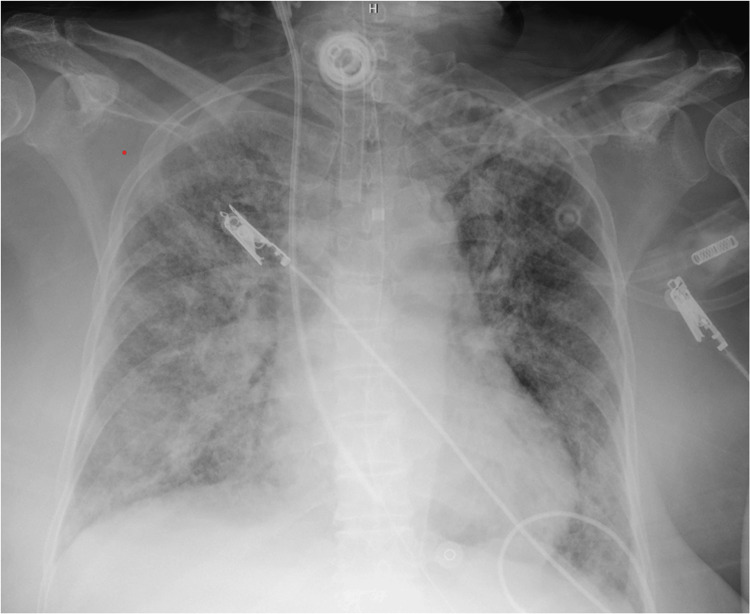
Portable anteroposterior chest radiograph obtained after veno-venous extracorporeal membrane oxygenation (VV-ECMO) decannulation demonstrating persistent diffuse bilateral pulmonary opacities.
